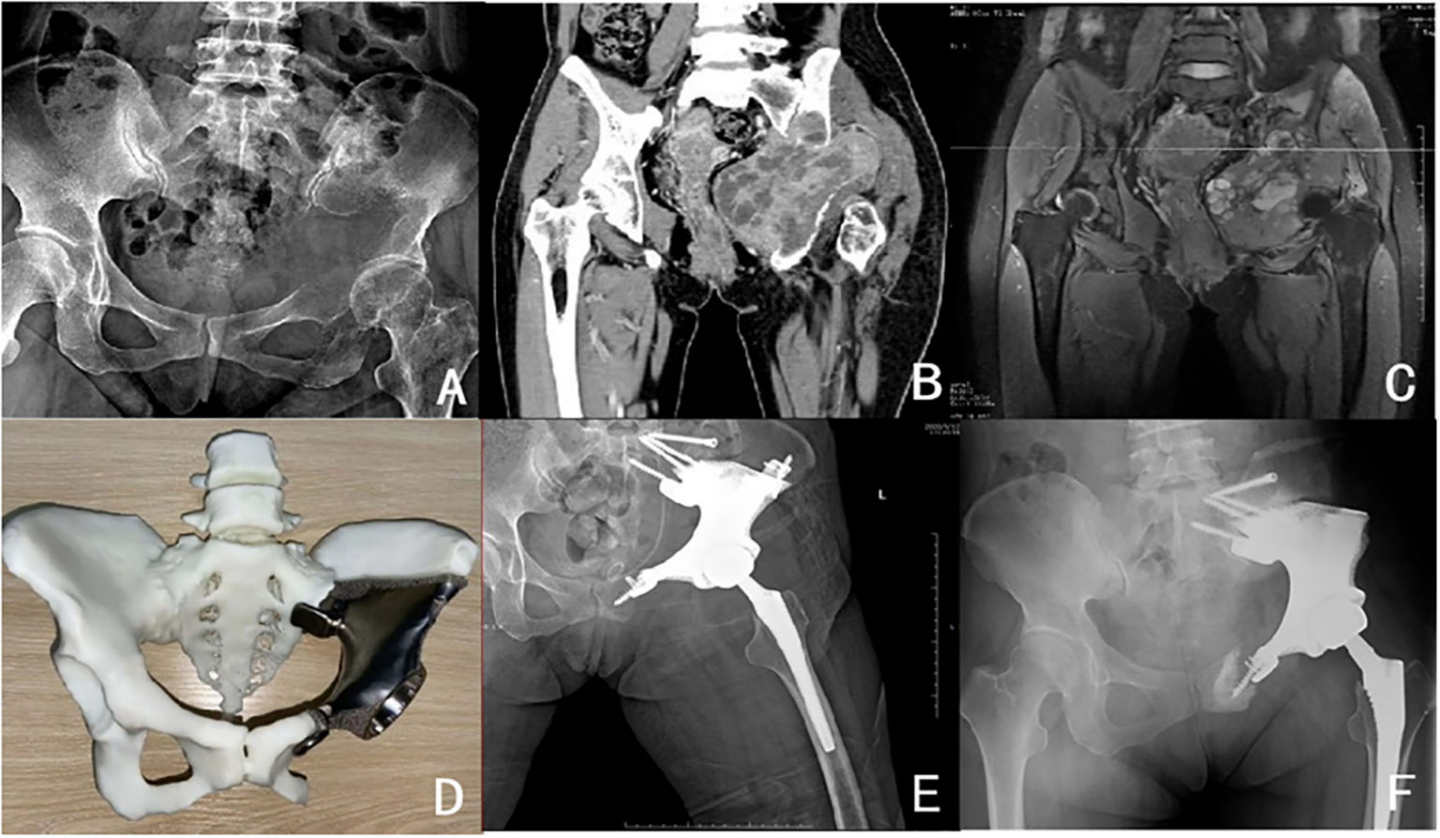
Patient,female,36 years old,pathology of malignant giant cell tumour of bone (A) Preoperative pelvic X-ray; (B) Preoperative pelvic CT; (C) Preoperative pelvic MRI; (D) Preoperative pelvic 3D printed tumour prosthesis; (E) Postoperative review pelvic X-ray; (F) Postoperative loosening of symphysis pubis fixation device.
